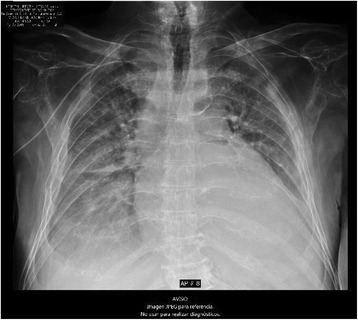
Chest X-ray on day 17. Increased cardiac silhouette
